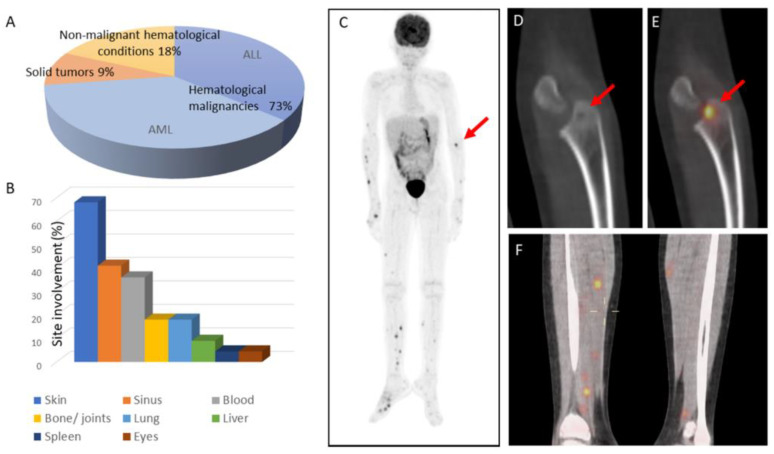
Invasive fusariosis in children: patient characteristics and infection patterns. (A) Distribution of underlying conditions in the study cohort. (B) Sites of involvement. (C) Coronal PET-CT of an 11-year-old boy with AML and disseminated fusariosis, with multiple cutaneous, intramuscular, and skeletal fluorodeoxyglucose (FDG)-avid lesions. A lytic lesion in the left radius is indicated by the arrow. (D) CT of a left radial lytic lesion (arrow) (E) with pathological FDG uptake (arrow). (F) Coronal lower limb PET-CT showing multiple bilateral intramuscular calf lesions with FDG uptake.
